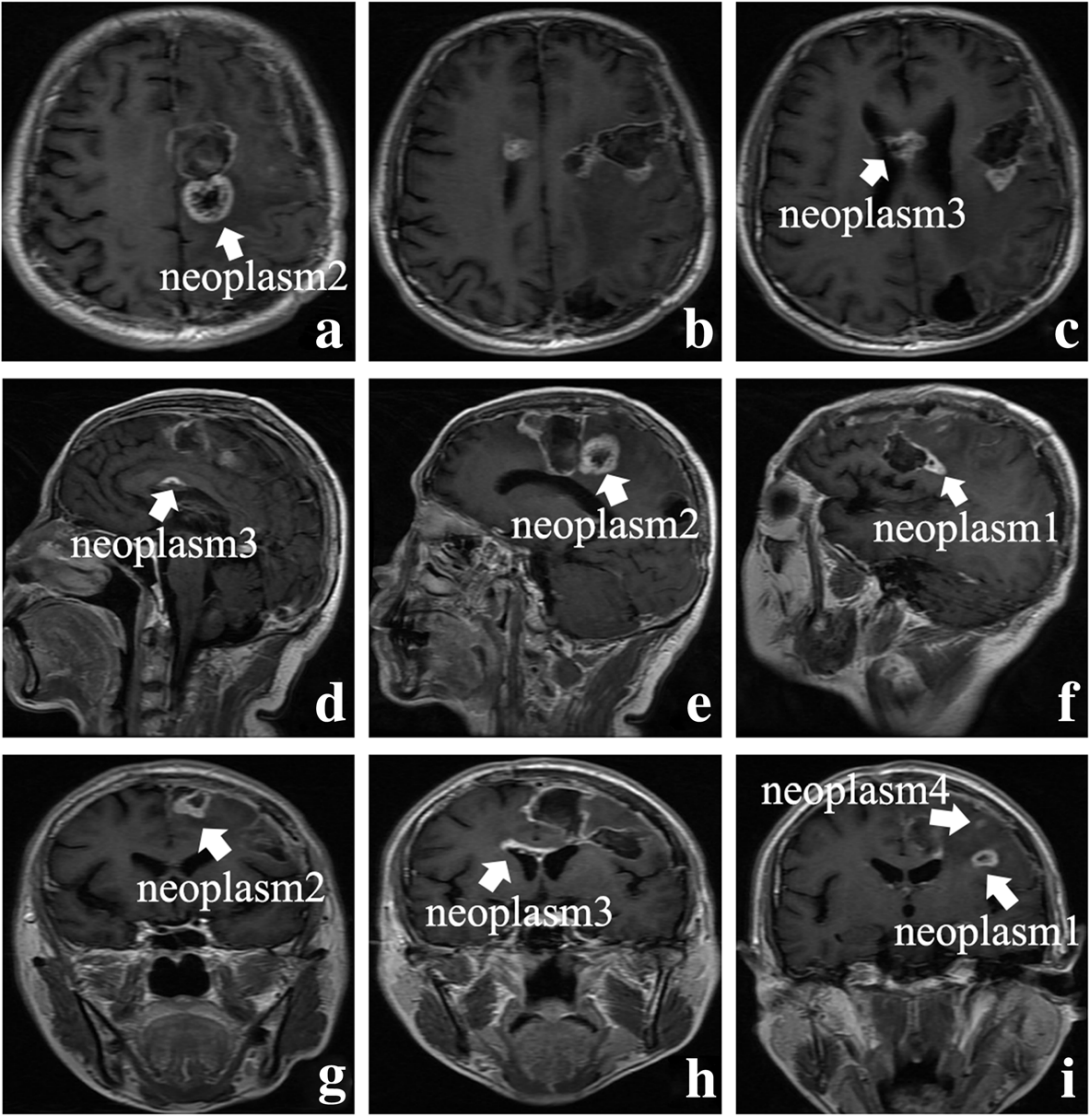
The axial, sagittal, and coronal contrast-enhanced MRI images after 2 cycles of chemotherapy (a–i). Former two new lesions enlarged distinctly; two more new lesions had emerged again (arrows represent the neoplasms)
